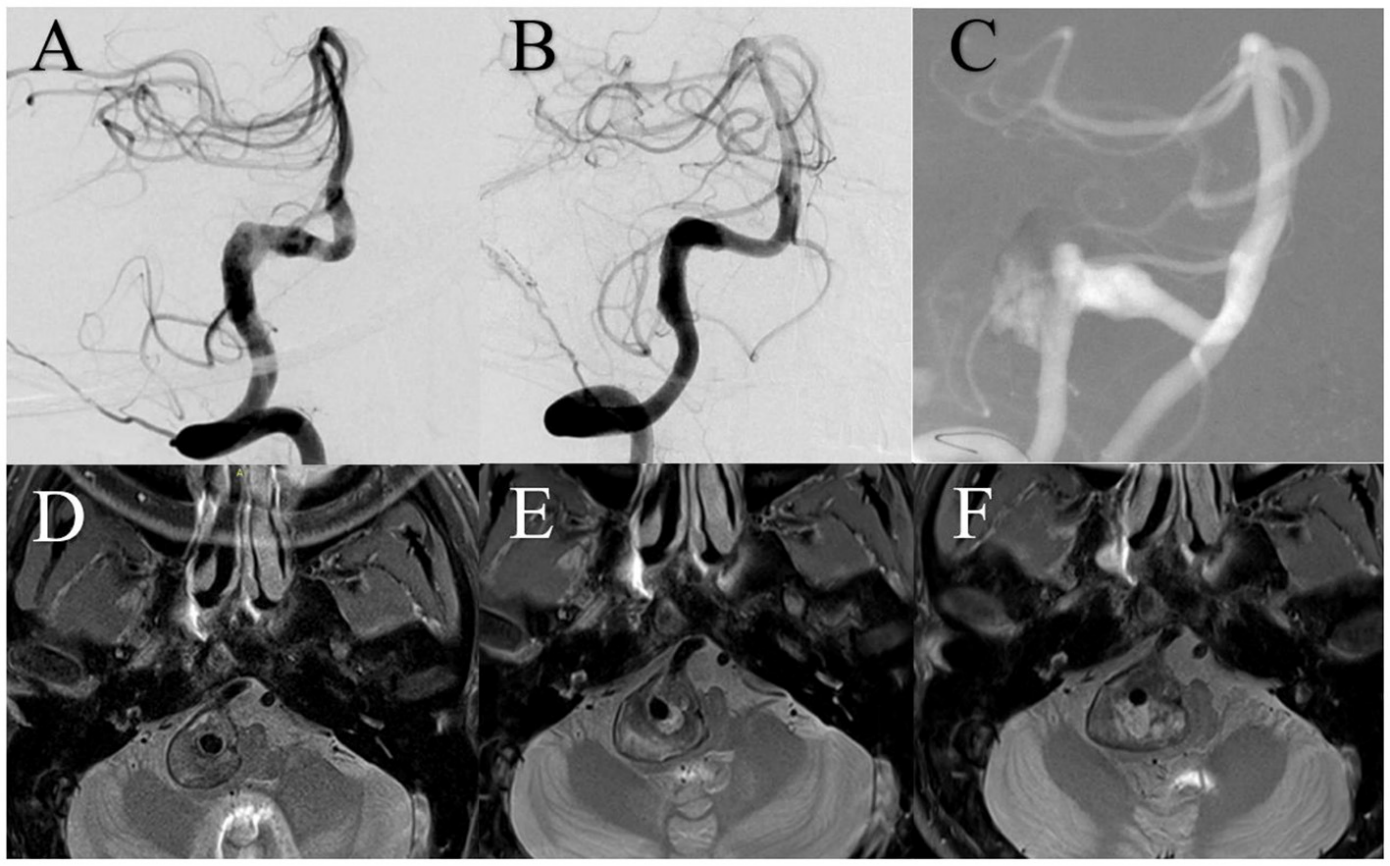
(A) Digital subtraction angiography (DSA) showed the right vertebral artery aneurysm. (B) DSA immediately after treatment showed satisfactory arterial reconstruction. (C) DSA 2 years after treatment showed aneurysm recurrence. Magnetic resonance imaging demonstrated intramural hematoma growth from before treatment (D) to 1 year (E) and 2 years (F) after.
